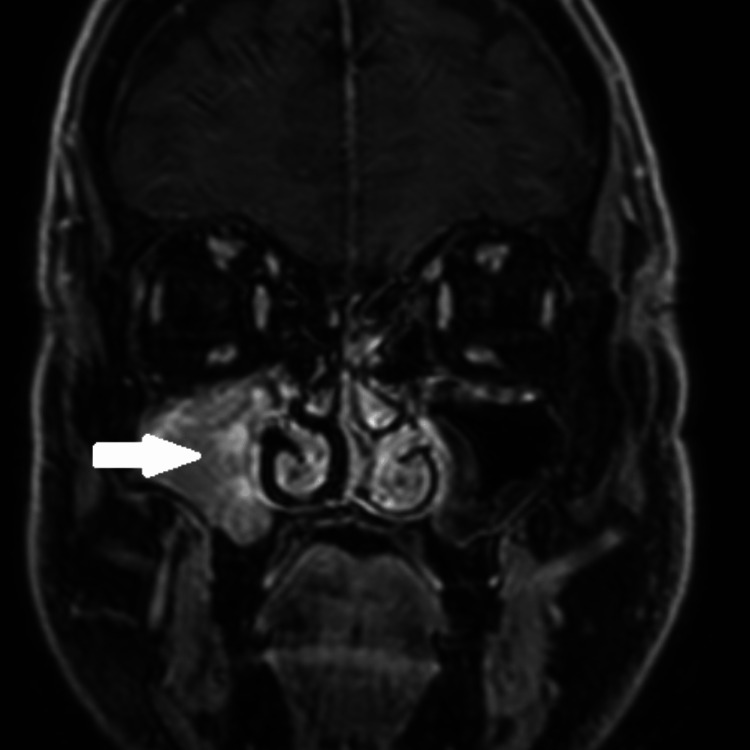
Pre-operative coronal contrast-enhanced MRI-T1 fat-saturated image at the level of maxillary sinus showing the same lesion restricted to the interior of the right sinus, with avid and slightly heterogeneous enhancement (white arrow).
